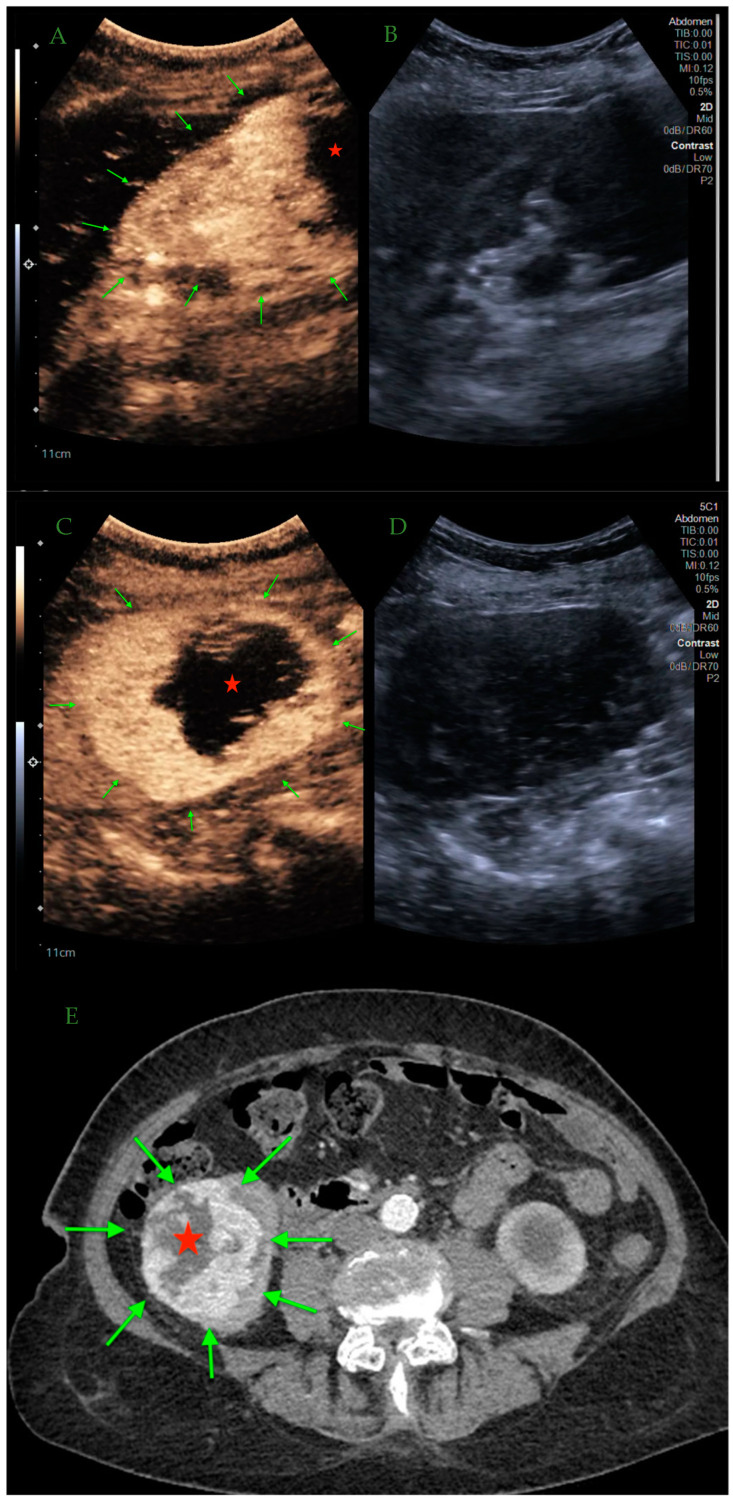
CEUS—RCC. Rapid, disorganized pattern of hyperenhancement of the RCC (green arrows) in the early arterial phase with marginal unenhanced region of necrosis (red star) (A,B). Clear boundaries of the lesion (green arrows) with a large area of internal necrosis (red star) can be appreciated at least as well as in CE-CT (C–E) [own source].
